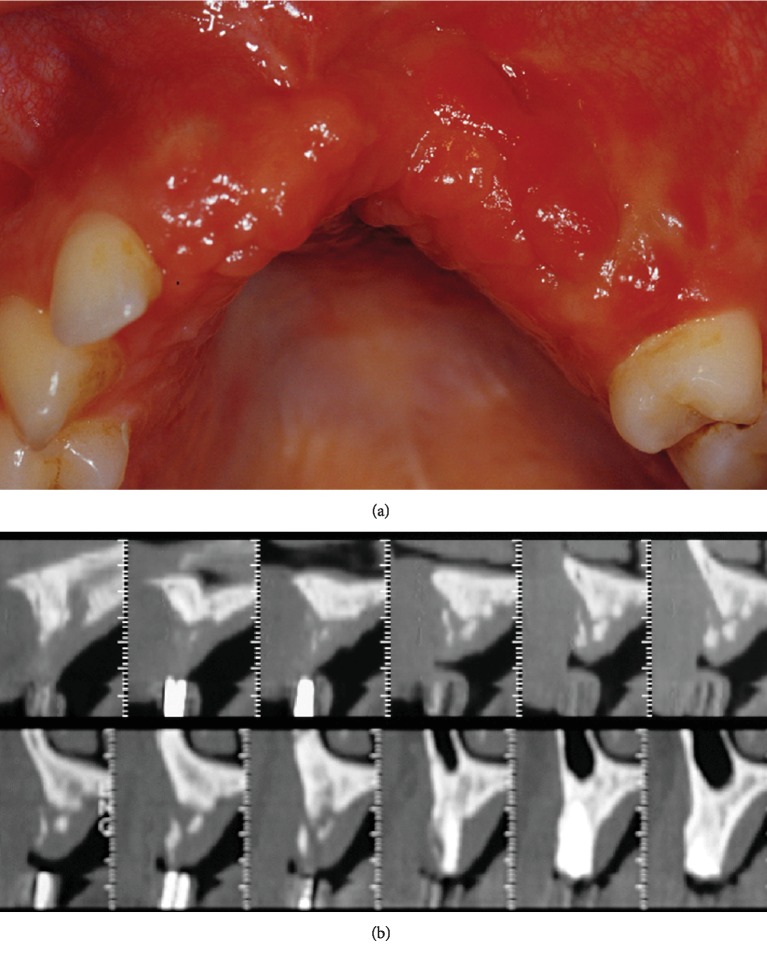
After two months of uneventful healing: (a) clinical view and (b) computed tomography scans showing the three-dimensional ridge defect and height of the available bone (5 mm) in the implant sites indicated by the template radiopaque markers.
